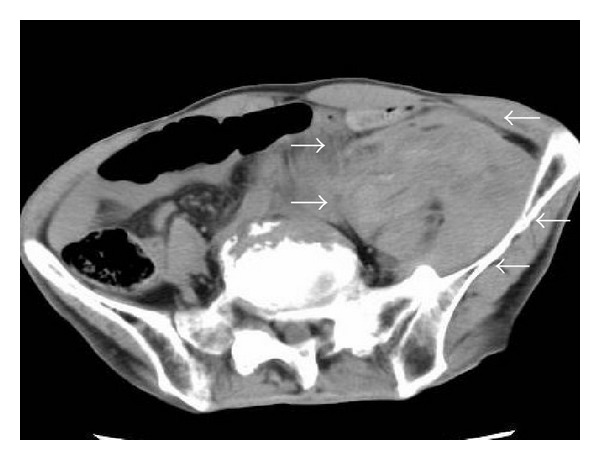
An abdominal CT showed about a 6 cm hematoma in the retroperitoneal space (arrow).
